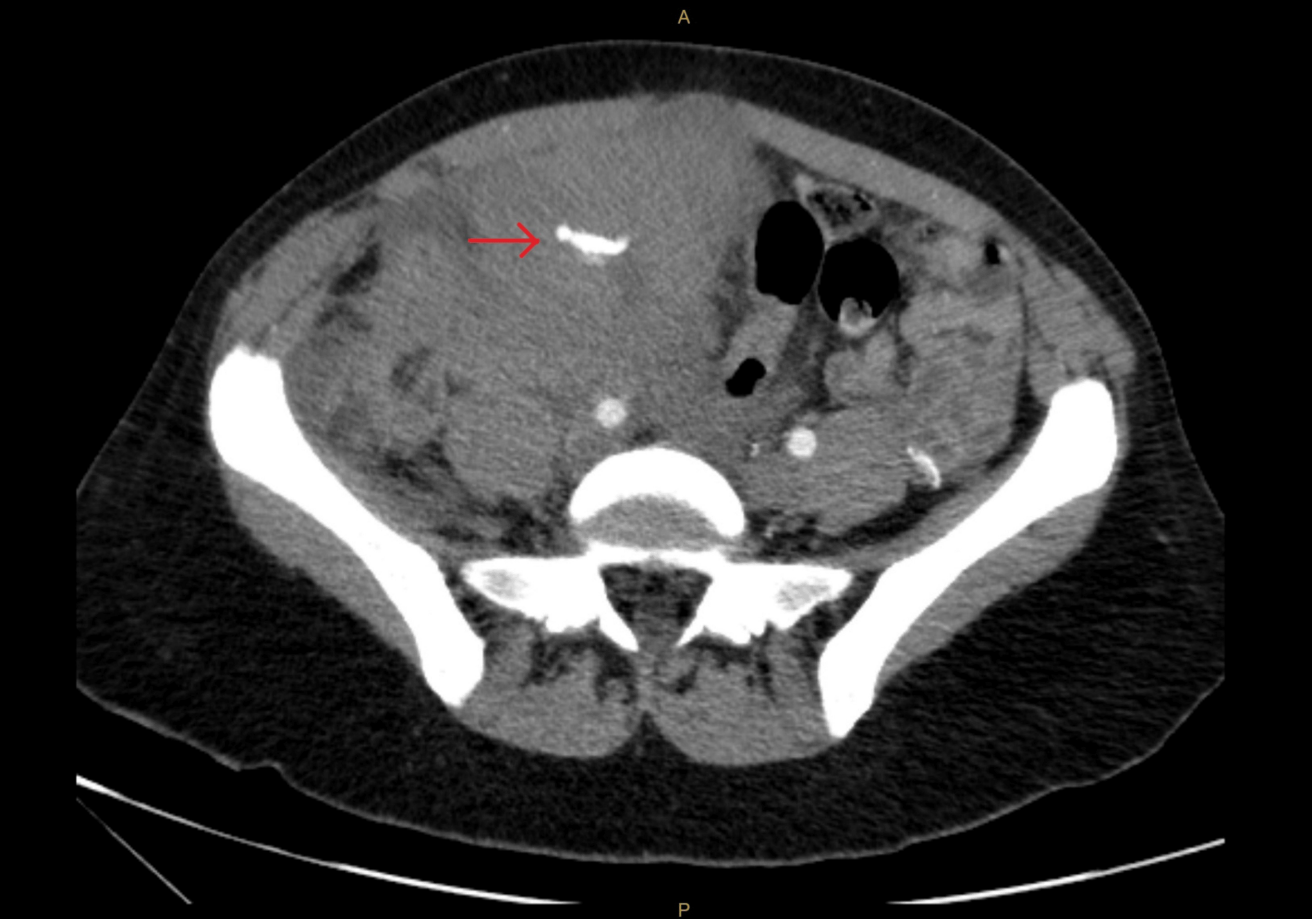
Transverse view of the initial CT angiogram, with the red arrow showing the aberrant artery running through the hematoma.
